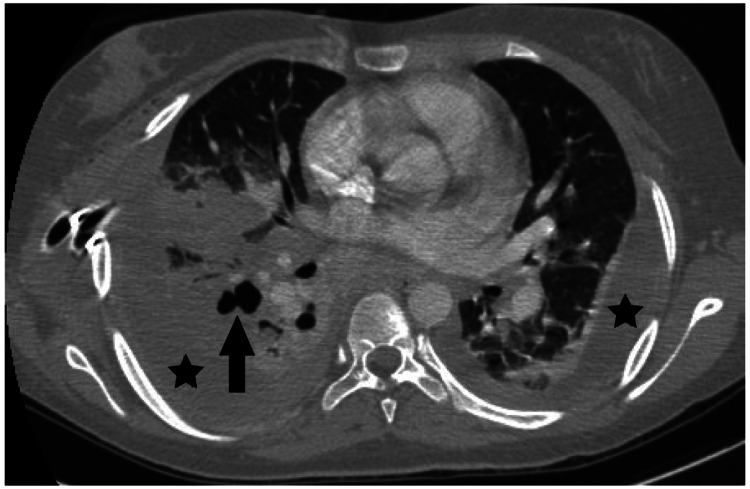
Chest computed tomography image (transverse view). The image shows bilateral pleural effusion (black stars) features of necrotizing pneumonia (multiple pneumatoceles: arrow).
